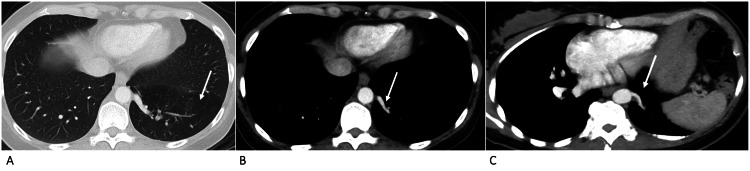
Case 2 - axial images of contrast-enhanced CT of the thorax Axial images of contrast enhanced CT of the thorax in lung window (A), mediastinal window (B), and reformatted maximum intensity projection mediastinal window (C) demonstrates pulmonary sequestration in the posterior segment of the left lower lobe seen as hyperinflation (arrow in A) and supplied by an arterial branch arising directly from descending thoracic aorta (arrows in B and C).
